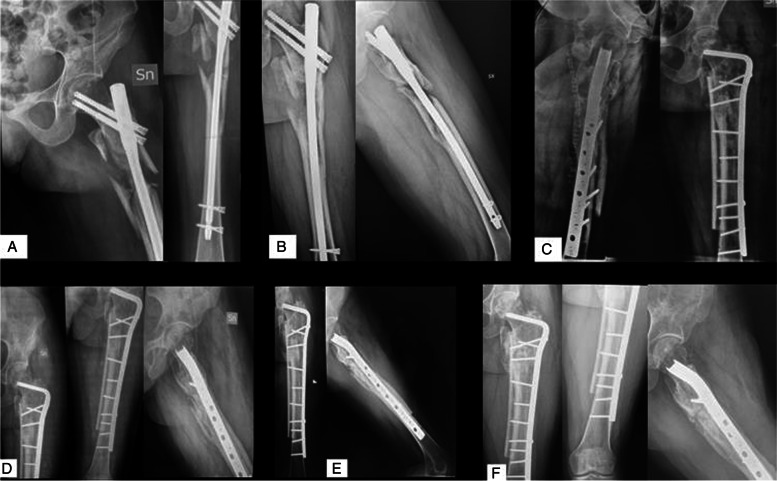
A AP and LL radiograph of femur subtrochanteric fracture of a 22 years old girl, due to a high energy trauma; B AP and LL radiographs at 6 months follow-up, showing a hypertrophic non-union; C AP and LL of the reintervention with blade plate, medial strut allograft and blade plate failure after 3 months; D AP and LL radiograph of postoperative at 2 months follow-up; E AP and LL radiograph at 4 months follow-up; f) AP and LL radiograph at 9 months follow-up
